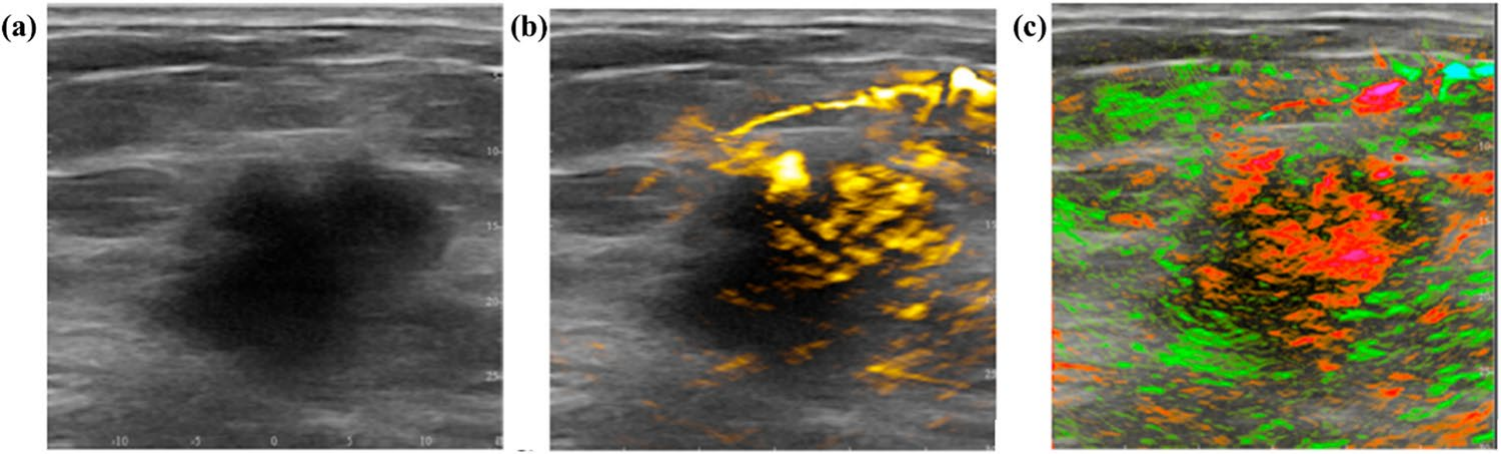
The example of a breast carcinoma OA/US imaging: a a 2.6-cm malignant mass on grayscale ultrasound; b the overlaying US with OA imaging, which illuminates increased internal total hemoglobin; c the overlaying US with OA imaging, which illuminates diffuse internal blood deoxygenation. The figure is reproduced with the kind permission from [96]
